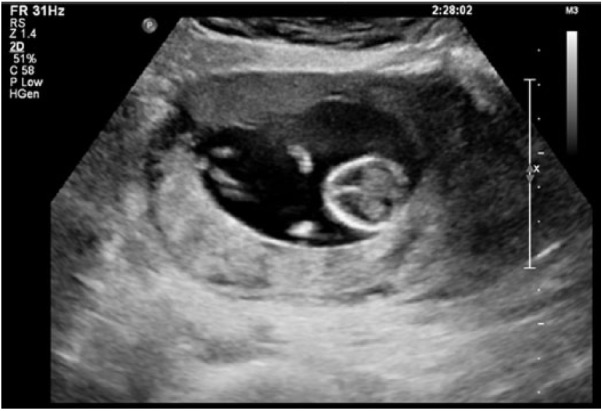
Transvaginal ultrasound with the live intramural pregnancy seen.
